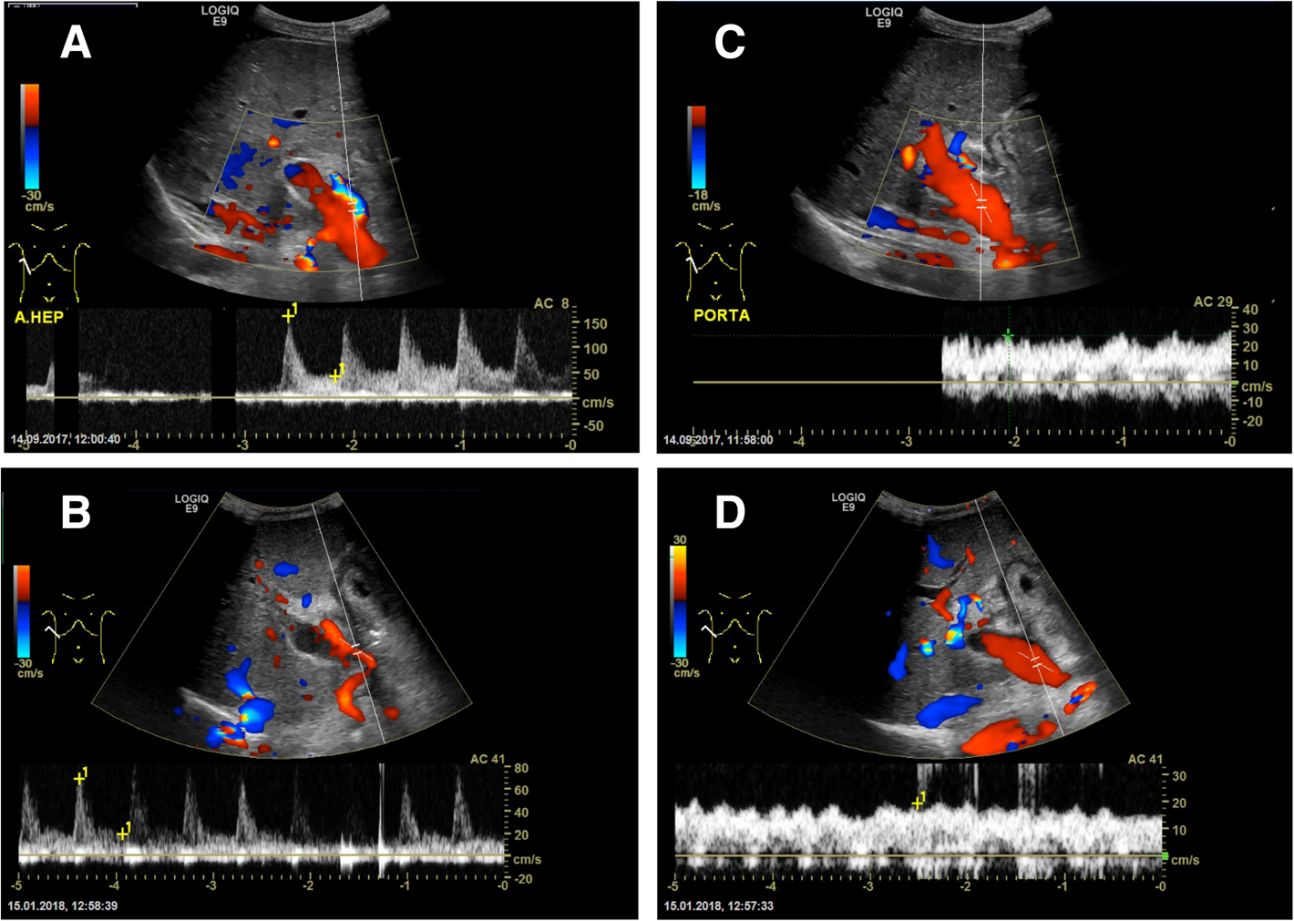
Circulation of the liver graft. The pictures to the left show examination of the common hepatic artery of the liver graft with US Doppler before (a) and after (b) CRS-HIPEC with a resistive index (RI) of 0.73 and 0.72 respectively. The pictures to the right show US Doppler examination of the portal vein of the liver graft before (c) and after (d) CRS-HIPEC with no resistance found in either exam
